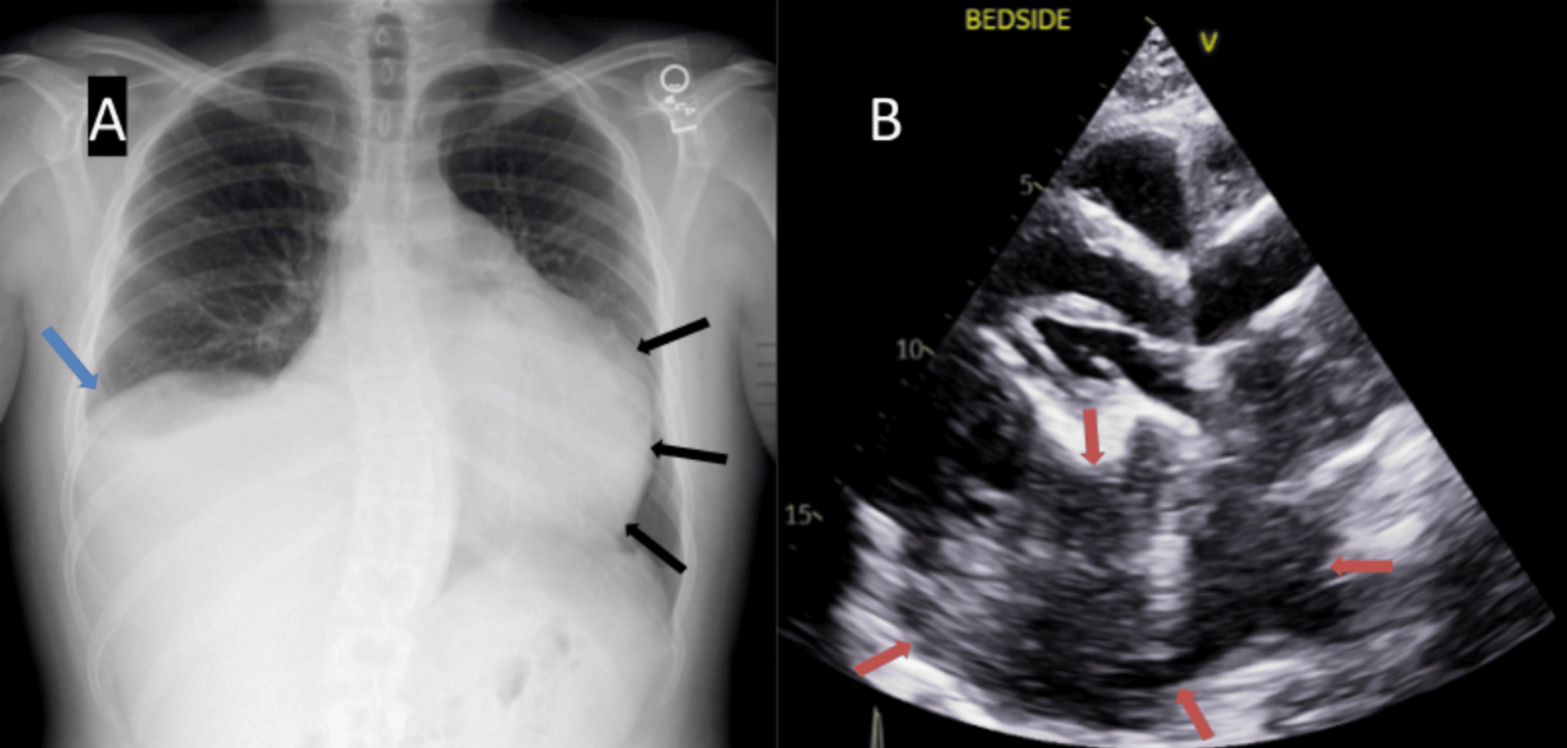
(A) Radiograph of the chest (PA view) with cardiomegaly (black arrows in A) and right small pleural effusion (blue arrow in A). (B) Bedside echocardiogram with large posterior pericardial mass (orange arrows in B). PA: posteroanterior
